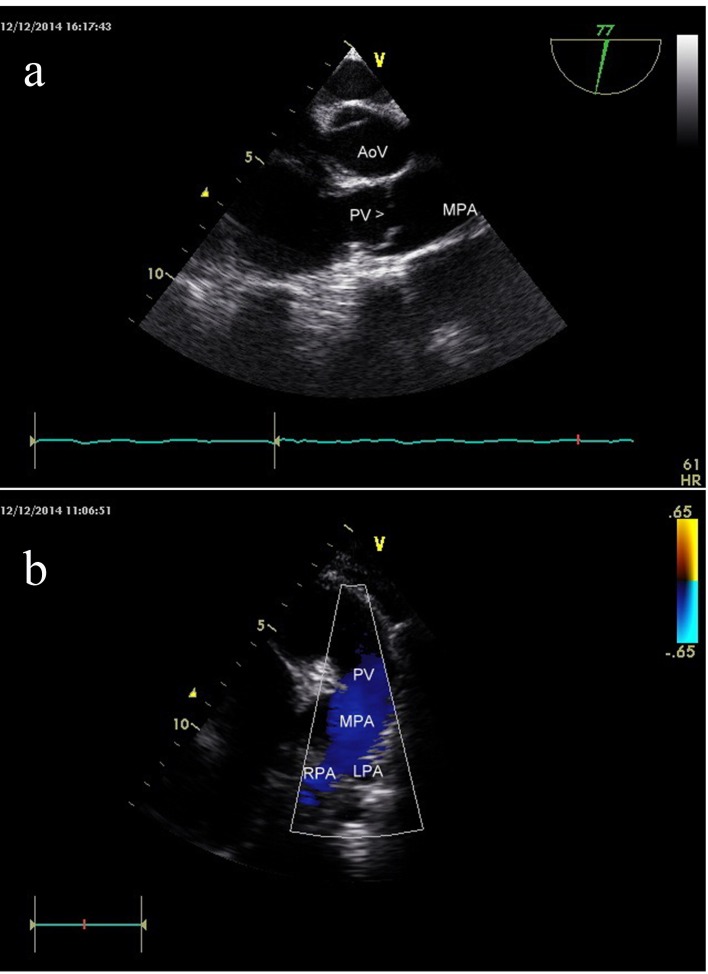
(a) Transesophageal echo showing patent pulmonary valve and pulmonary artery. (b) Transthoracic echo showing patent pulmonary valve and pulmonary artery.
